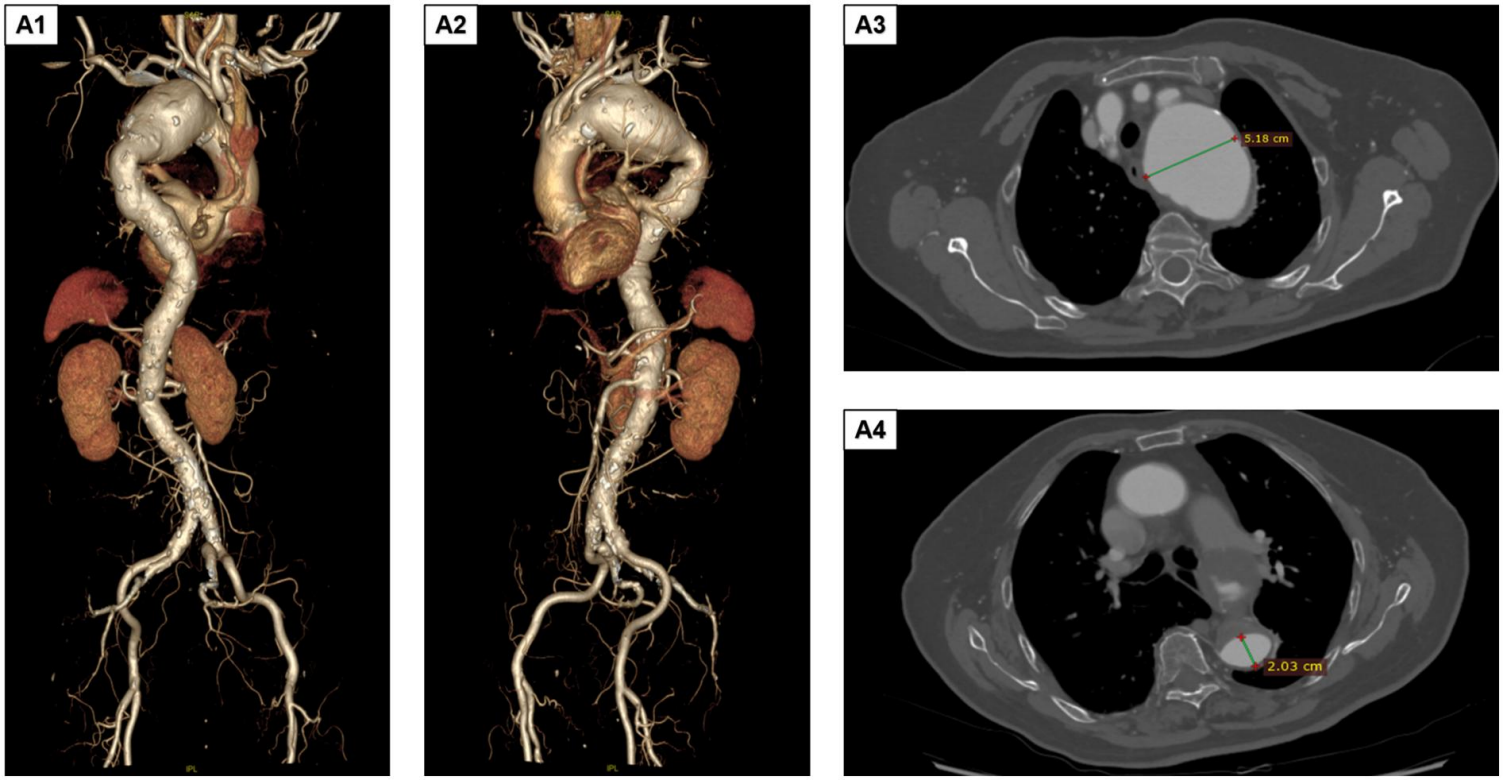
Preoperative DSA and CTA findings. (A1,A2) The aortic arch aneurysm involves the left subclavian artery and extends downward to the thoracic aorta. The size of the aneurysmal sac is approximately 9.0 × 5.1 cm. (A3,A4) The aortic arch shows aneurysmal dilation, with the maximum diameter measuring approximately a5.18 cm. Additionally, aneurysmal dilation of the thoracic aorta is observed, with a diameter of approximately 2.03 cm. DSA, digital subtraction angiography; CTA, computed tomography angiography.
